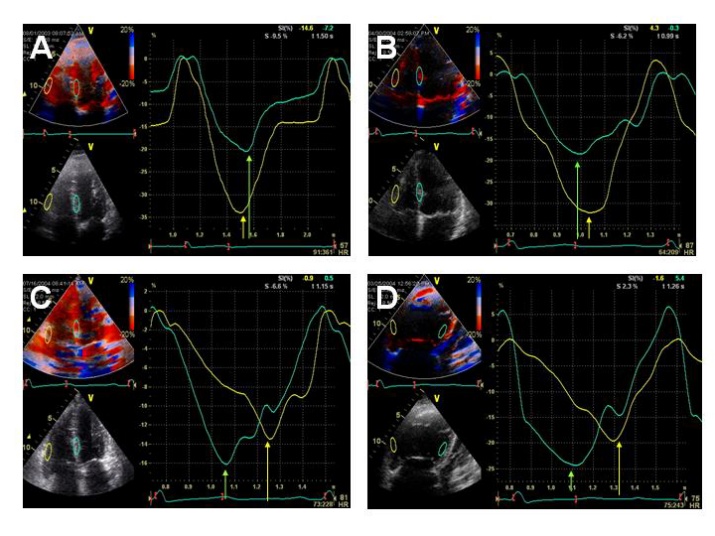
A representative tissue Doppler image is shown demonstrating (A) Almost synchronized time-to-peak longitudinal peak strain of both right ventricular free wall (yellow curve) and ventricular septum (green curve) in an individual without PAH. (B) Slightly delayed time-to-peak longitudinal right ventricular free wall peak strain (yellow curve) when compared to ventricular septum peak strain (green curve) in a patient with mild PAH. Note that there is no significant reduction in RVw strain generation. (C) A more noticeable delayed time-to-peak longitudinal right ventricular free wall peak strain (yellow curve) when compared to ventricular septum peak strain (green curve) is now evident in a patient with moderate PAH as well as in (D) in a patient with severe PAH and severe RV dysfunction. Note that in both (C) and (D) there is a significant reduction in RVw strain generation.
